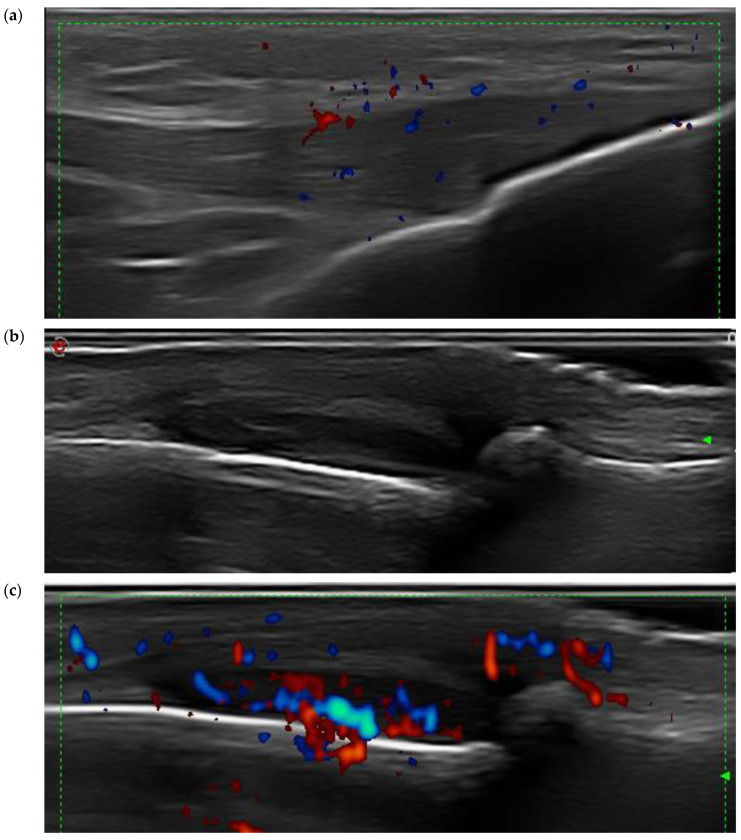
(a) PsA patient within treatment target: grayscale (GS) and power Doppler (PD) examination, longitudinal scan of the distal patellar tendon. Abnormal thickening and hypoechogenicity of the enthesis and the presence of PD are noticed. (b) PsA patient within treatment target: GS (b) and PD examination (c), longitudinal scan of the dorsal side of finger 5. Active PD synovitis at the PIP level and active paratenonitis of the extensor of the fifth finger are observed (Esaote MyLab Twice ultrasound device; 18 MHz linear frequency probe).
